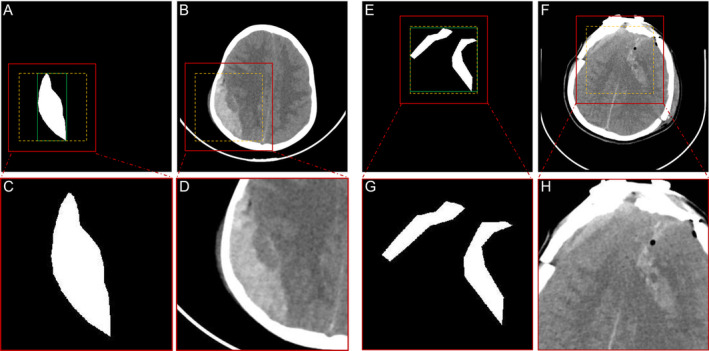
Examples of annotated images. (A) and (E): GT of input segmentation Stage I; (B) and (F): CT slices of input segmentation Stage I; (C) and (G): Mask of input segmentation Stage II; (D) and (H): ROI squares of input segmentation Stage II.
